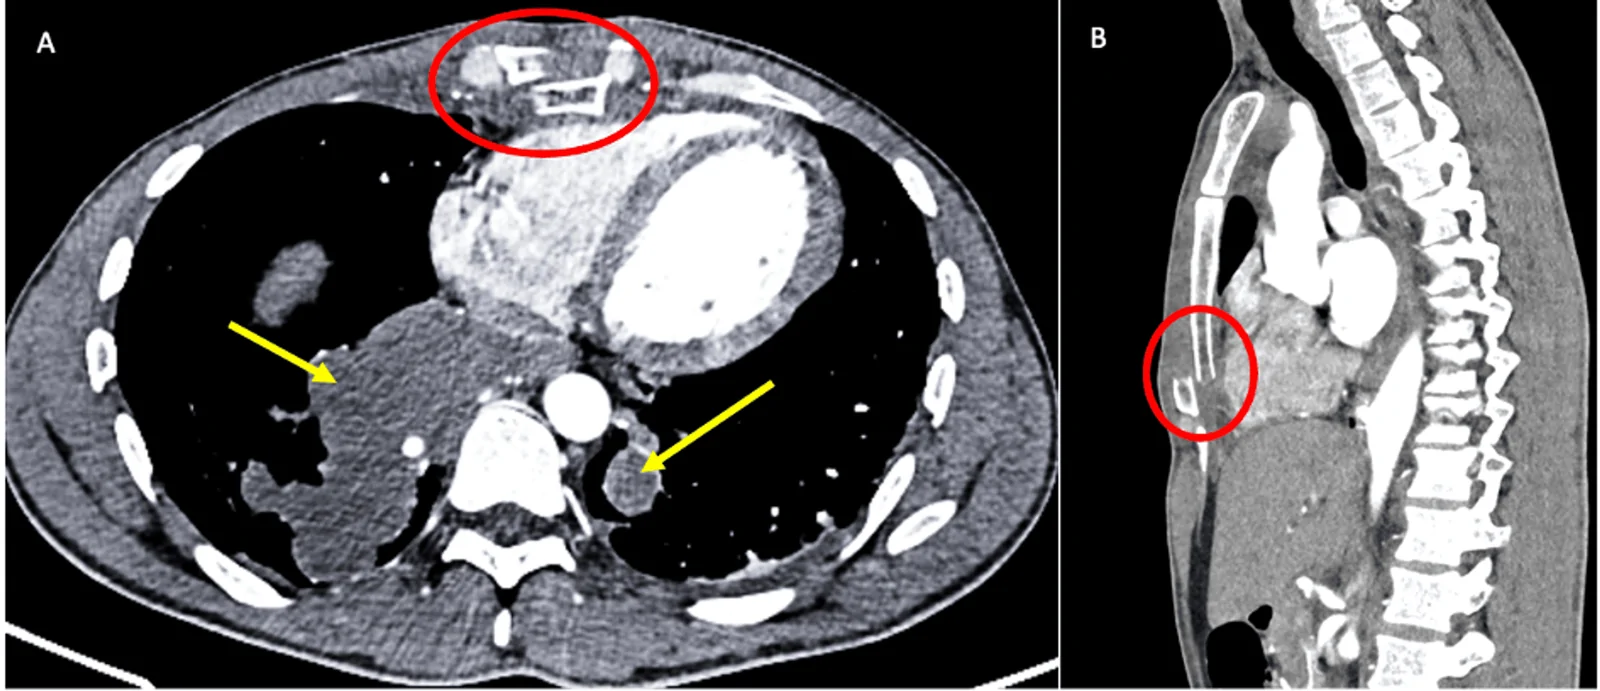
Axial (A) and sagittal (B) computed tomography (CT) angiography images showing the displaced sternal fracture (red circle) and heterogenous tissular and cystic bilateral lower lobe consolidation, larger in the right side (yellow arrows).
